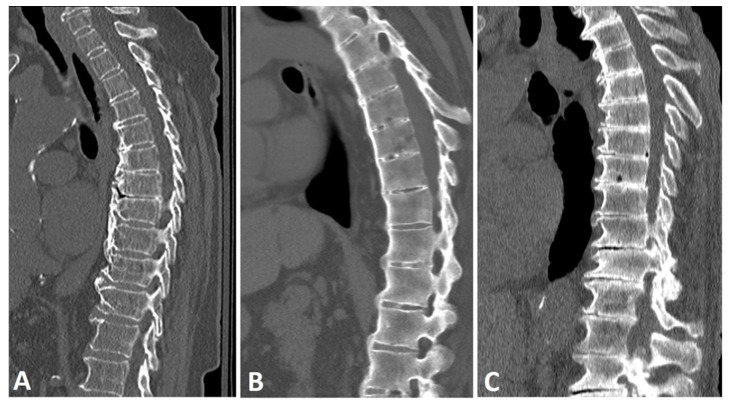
Sagittal CT reconstructions of the thoracic spine in three different patients: (A) a patient with DISH with thick, flowing, horizontally oriented, coarse osteophytes; (B) a patient with AS with slender, vertically oriented syndesmophytes; and (C) a patient with degenerative disc disease with horizontally oriented, non-flowing osteophytes. Note that the disc space is relatively preserved in the DISH subject, but not in the patient with a degenerative spine.
